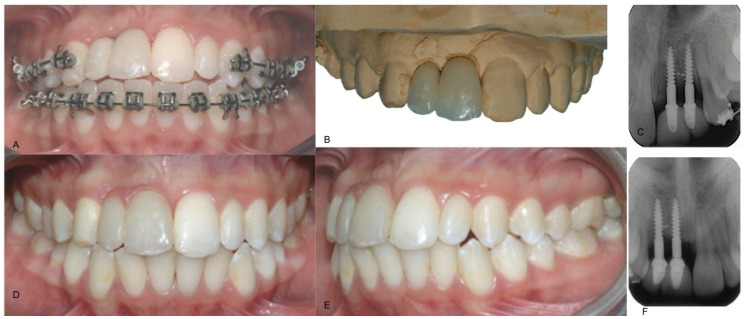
(A) Provisional resin crown on the implant, (B) plaster model of provisional crown, (C) periapical X-ray control after provisional crown placement, (D) frontal view of definitive crown after 13 years, (E) lateral view and (F) periapical X-ray control after 13 years.
